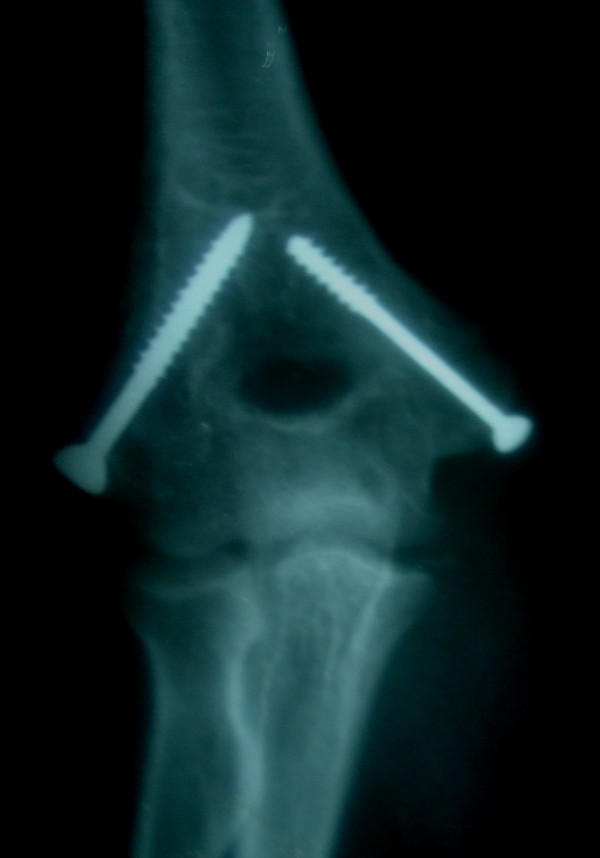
post operative radiograph Ap view.
